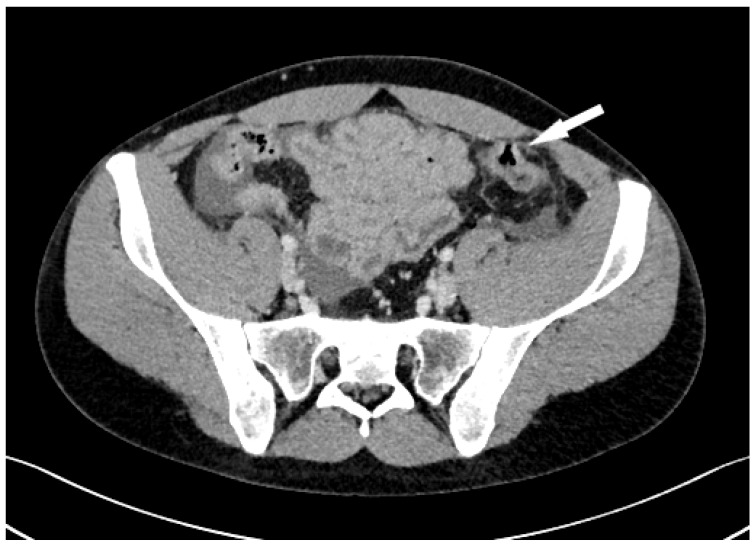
Abdominal CT shows sigmoid colon perforation (arrow).
